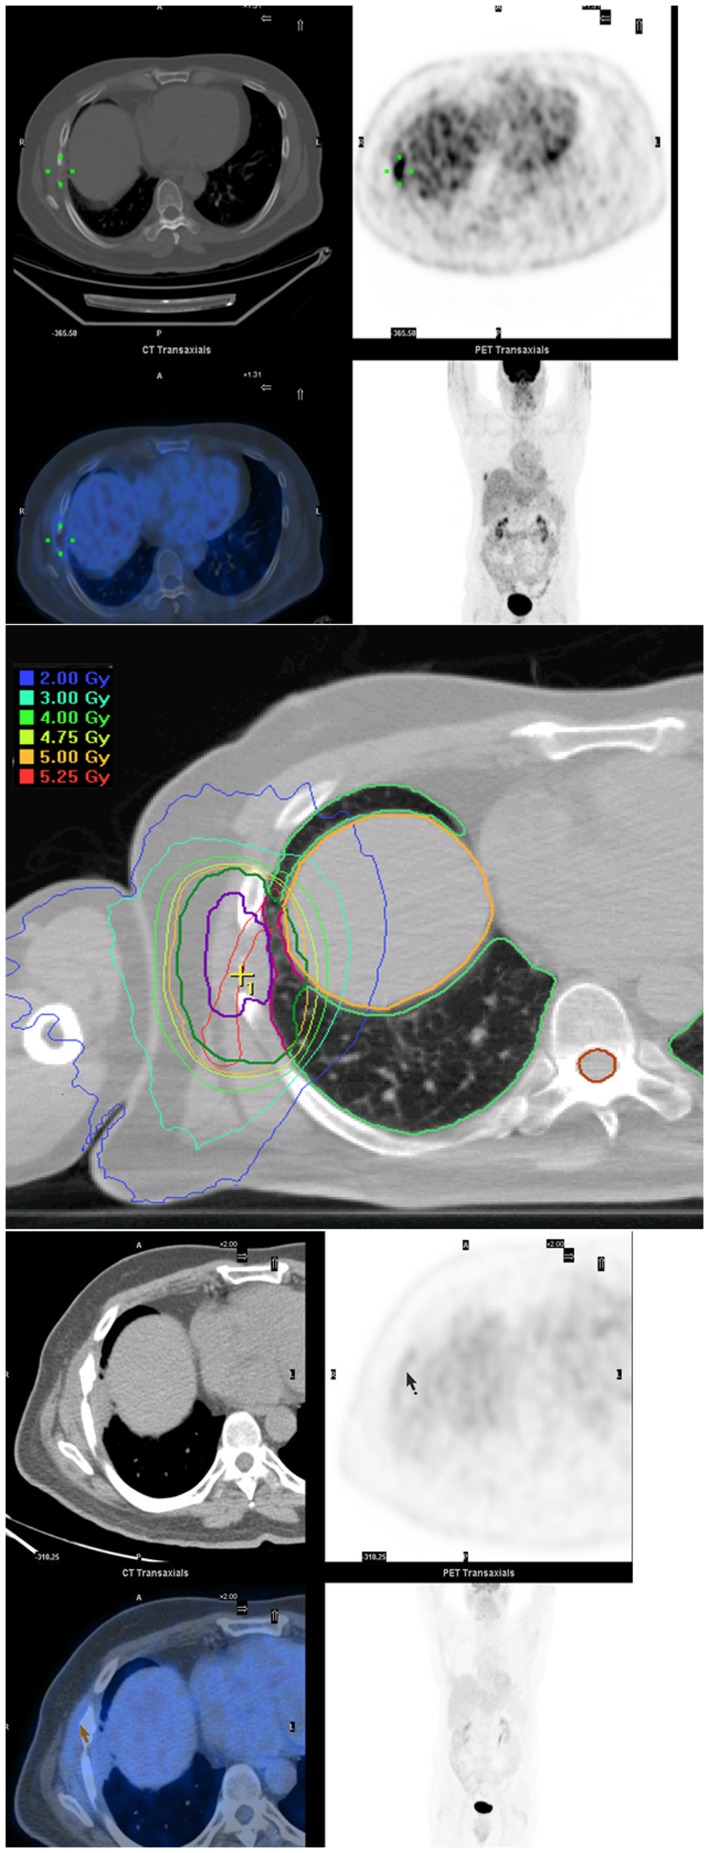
Representative patient treated with concurrent sunitinib and IGRT. a) Pretreatment PET/CT demonstrates a biopsy proven solitary metastasis in the right 7^th^ rib in a patient with non-small cell lung cancer. b) The rib lesion was treated with Novalis using dynamic arcs using the ITV method with an abdominal belt used to dampen respiratory motion. Daily kV imaging was accomplished using bone fusion. There was excellent coverage of the PTV with selective sparing of the normal lung, liver and skin. c) Complete response on PET/CT 23 months after Sutent +RT.
